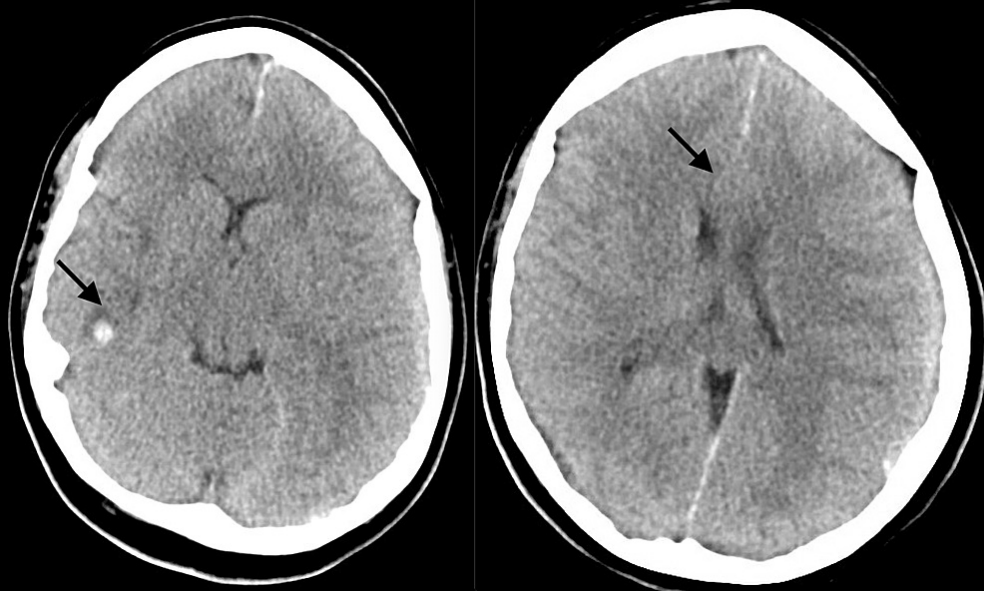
CT images with overall findings concerning for acute on subacute left hemispheric subdural hematoma Image on left: Arrow pointing to 6 mm hyperdense focus right temporal lobe inferiorly with adjacent vasogenic edema concerning for small parenchymal hematoma. Image on right: There is an extra-axial collection over the left frontoparietal region with maximal thickness of 6 mm and mass effect resulting in 4 mm left-to-right midline shift.
